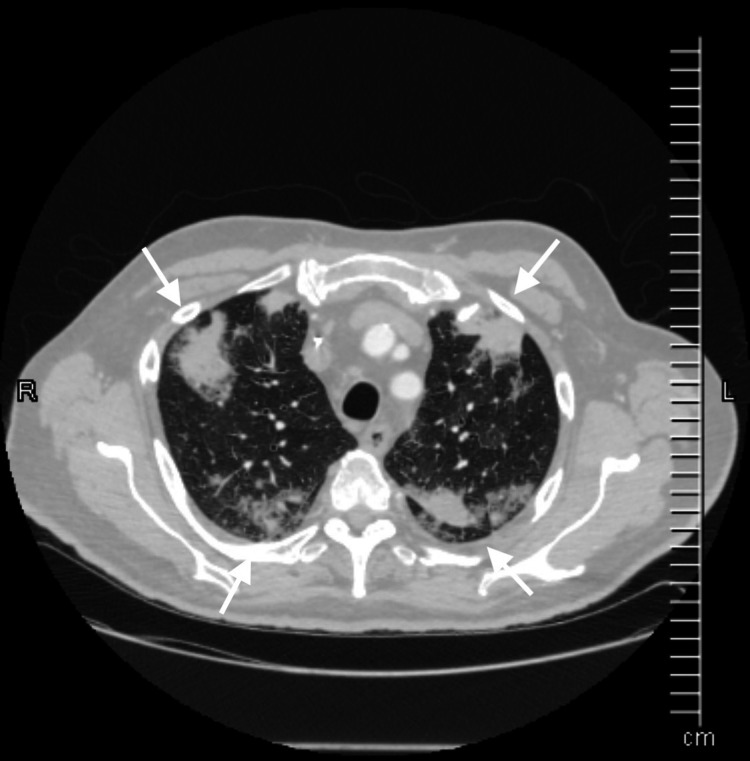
CT showing involvement of outer one-third of lung zones.
